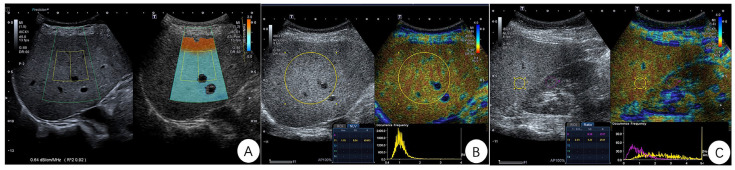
Representative images of the ATI, NLV, and Ratio examination in one patient. (A) ATI image: The ultrasound (US) image is shown on the left and the same US image overlaid with the attenuation map is shown on the right. The attenuation coefficient (AC) is shown to be 0.64 dB/cm/MHz, and the coefficient of determination (R^2^) is 0.92. (B) NLV image: A 50 mm ROI is placed in the liver parenchyma and 10 mm below the liver capsule. The average value (Ave) of NLV is 1.15 and the standard deviation (SD) is 0.54. (C) Ratio image: A 10 mm circular ROI is free of vessels or artifacts within the liver parenchyma, and a 10 mm circular ROI is placed within the right kidney cortex which is free of fat, large vessels and renal pyramids. The liver–kidney intensity ratio (Ratio) of 2.51 and SD of 1.27 are displayed at the bottom of the image.
